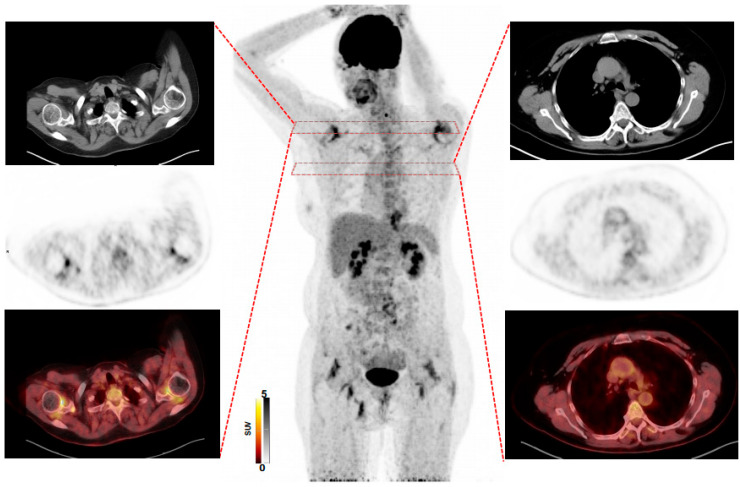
A 58-year-old woman with typical symptoms of polymyalgia rheumatica, including shoulder and hip girdle pain. Maximum intensity projection (middle) revealed intense uptake in multiple joints and in the thoracic aorta. Transaxial CT, PET and PET/CT revealed intense uptake in the AC joints (left) and aorta ascendens (right), suggestive for combined polymyalgia rheumatica and giant cell arteritis.
